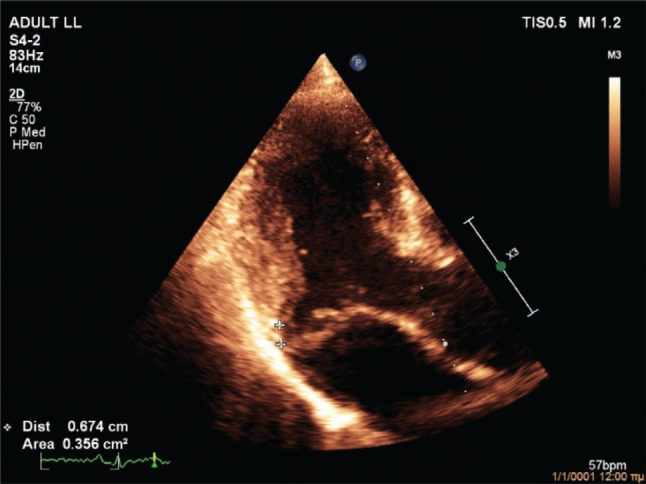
Case 2 echocardiogram showing mitral annular disjunction.
